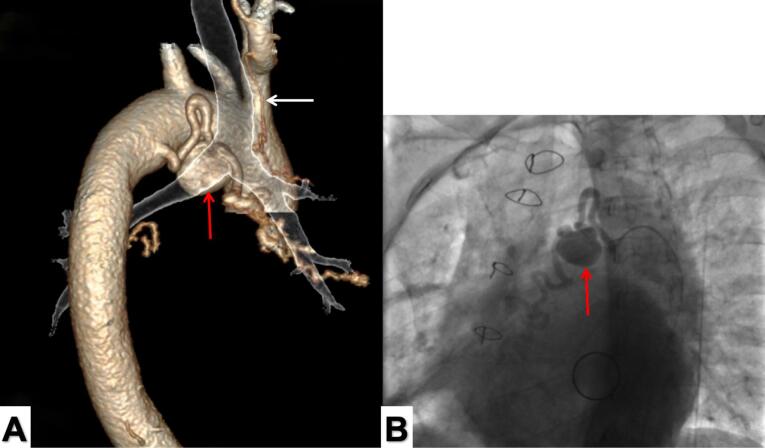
The patient underwent a successful anesthesia, right femoral artery puncture was performed and 6F sheath catheter was placed. We found the bronchial artery opening on the small curved side of aortic arch, with aneurysmal dilation extending and one outflow artery running to the pulmonary artery, Partial pulmonary artery visualization. In combination with the preoperative bronchial artery CT, a BAA with a bronchial artery-pulmonary artery fistula was considered. After exchanging the vascular sheath for support and changing to a C2 catheter, the bronchial artery was selectively entered. Angiography indicated a BAA measuring approximately 2.5x2.5cm (Figure 1B), with contrast medium flowing into the lower bronchial artery of the right lung. After several attempts with a guidewire and micro catheter, the bronchial artery aneurysm was successfully accessed, and embolization was performed with five coils, the outflow and inflow ends of the aneurysm were embolized with six additional coils (Figure 2). A follow-up angiogram showed no contrast filling in the main bronchial artery, the aneurysm, and the outflow end, confirming complete embolization (Supplementary File 1, Video 1).
